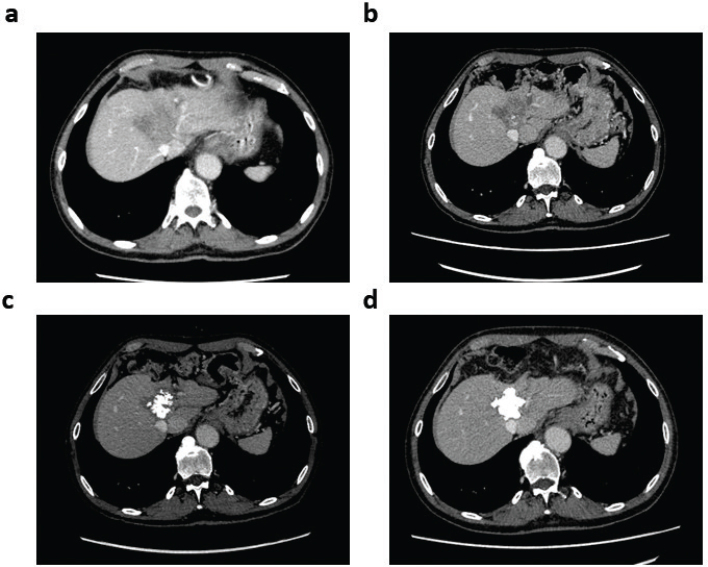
Tumor calcification in the patient with CCA on long-term pemigatinib treatment. Axial CT images show (a) stable disease after 13 cycles of GemCis chemotherapy, (b) 20% reduction in tumor size after three cycles of pemigatinib, (c) increasing tumor calcification after six cycles of pemigatinib, and (d) complete tumor calcification after approximately 3.5 years on pemigatinib. CCA: cholangiocarcinoma; CT: computed tomography; GemCis: gemcitabine + cisplatin.
